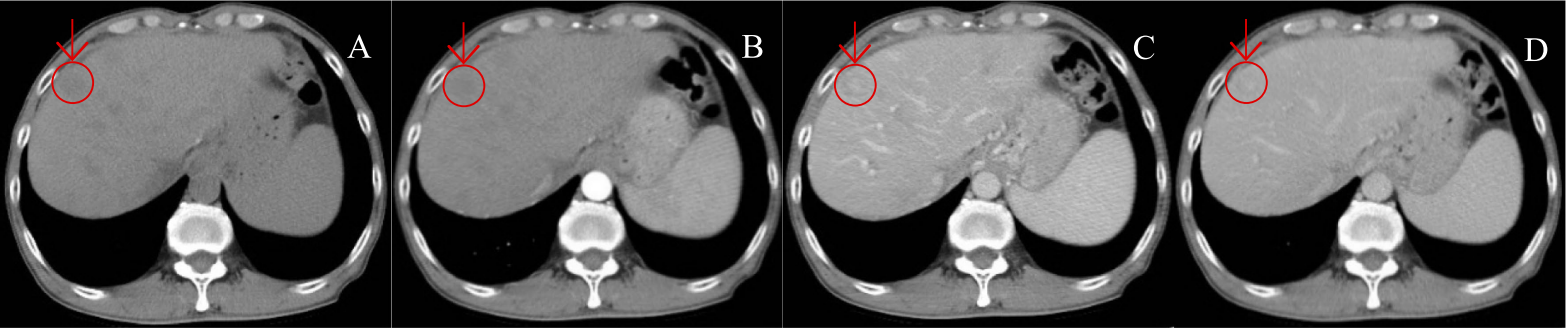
A patient with HCC was reviewed after TACE treatment. (A–D) show the images of CT plain (A) and enhanced scans of arterial phase (B), portal vein (C), and delayed phase (D), in sequence. CT plain scan: New lesions were seen in the hepatic parenchyma, with nodular hypodensity, the large one was about 19.6 mm in diameter; enhancement scan showed insignificant enhancement in arterial phase, slightly hypodense in portal vein and delayed phase, and hyperdense in the center, which was atypical of “fast-in-fast-out” performance.
